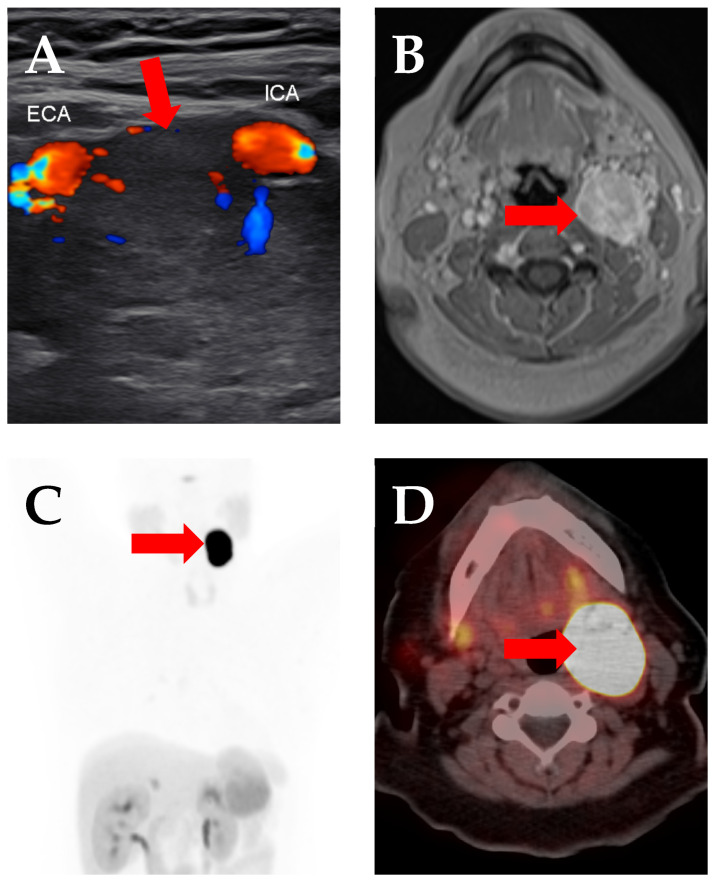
Images from a 46-year-old woman with a carotid body tumor. (A) Transverse ultrasound image demonstrates a heterogeneously hypoechoic mass (arrow) that is splaying the external carotid artery (ECA) and the internal carotid artery (ICA). (B) The mass was also imaged with axial, T1, contrast-enhanced MRI, where the significant vascularity of the tumor was reflected in avid enhancement (arrow). (C) Maximum intensity projection ^68^Ga-DOTATATE PET and (D) axial ^68^Ga-DOTATATE PET/CT images show intense uptake in the mass, suggesting a potential role for theranostics in such tumors.
